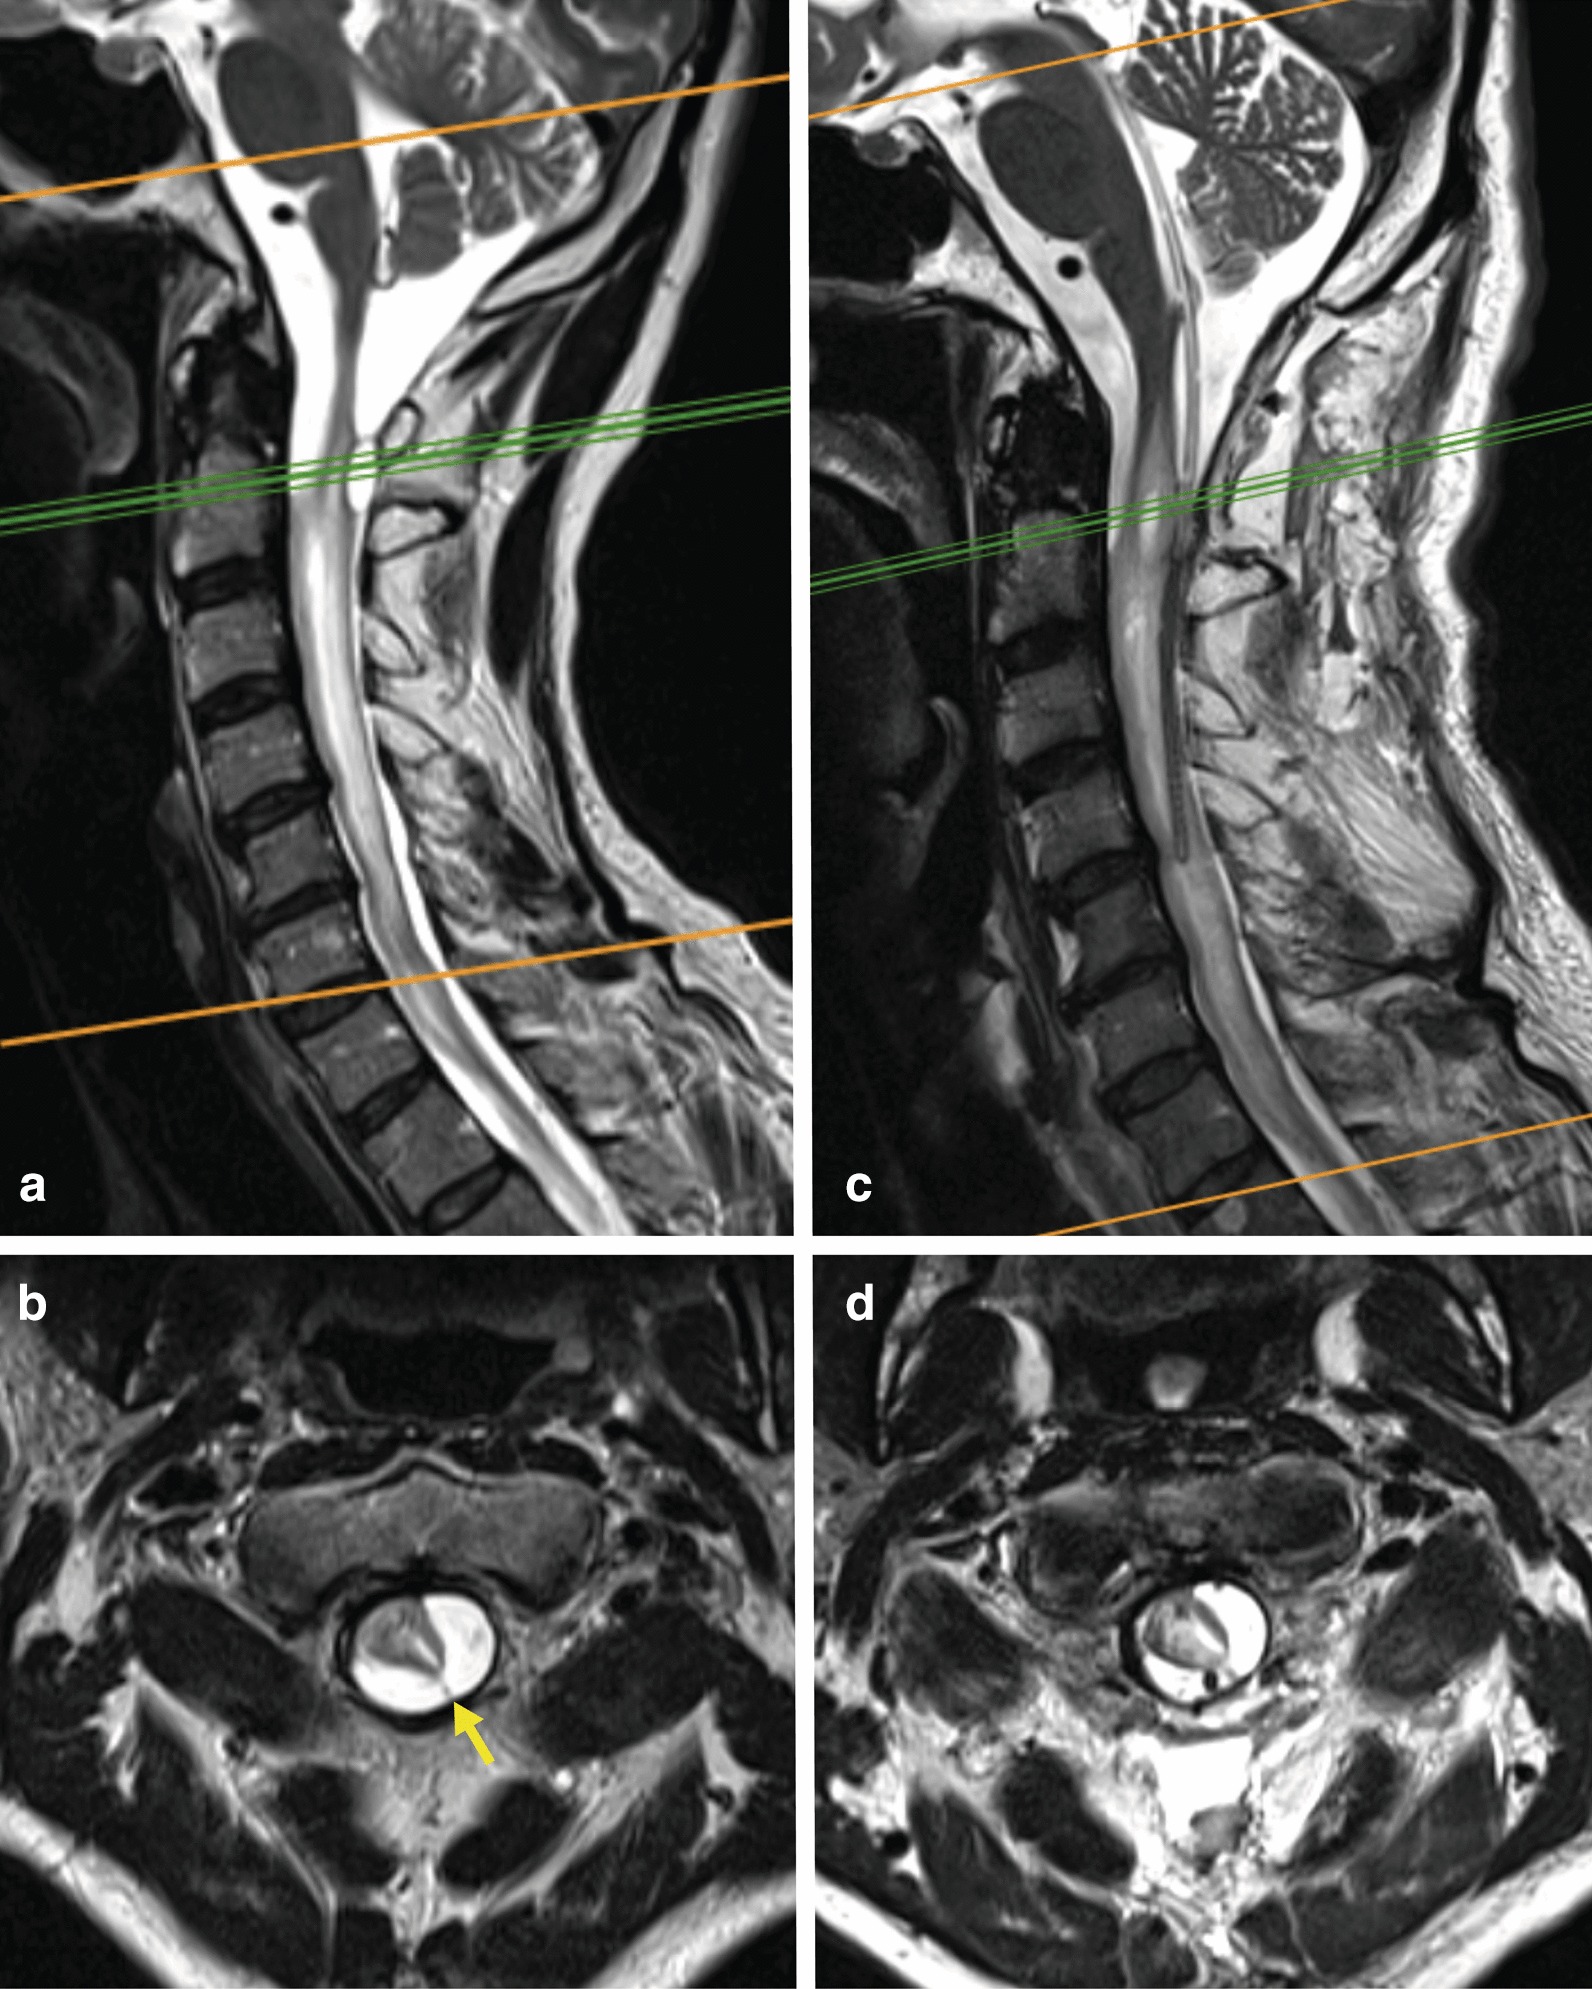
a, b T2-weighted magnetic resonance imaging (1.5 T, 3 mm slice thickness) 2 years post-injury demonstrated that the arachnoid cyst progressed and that a syrinx cavity developed while the medullary edema was still present. Arachnoid adhesions are marked by the yellow arrow. c, d The postoperative MRI following decompression, untethering, and drain placement revealed a minimal reduction of the arachnoid cyst but no resolution of the syrinx
